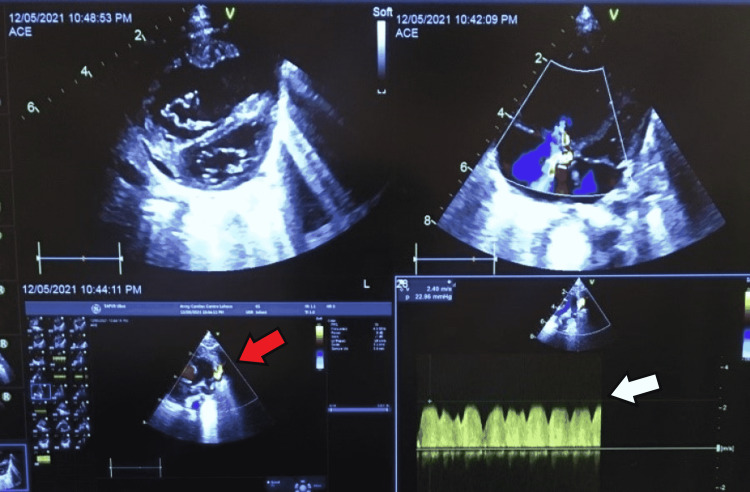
Pre-procedural transthoracic echocardiogram showing dilated right heart, moderate tricuspid regurgitation, and severe pulmonary hypertension. Pulmonary veins draining via ascending levoatrial cardinal vein (vertical vein) into the innominate vein with severe narrowing of the vertical vein (red arrow) and peak pressure gradient of 23 mmHg (white arrow).
